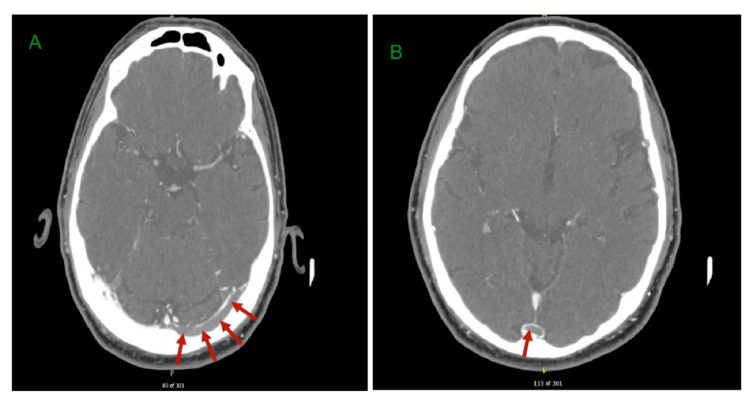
CT venograms. (A) Red arrows indicate a contrast-filling defect in the left transverse sinus, suggestive of thrombosis.
